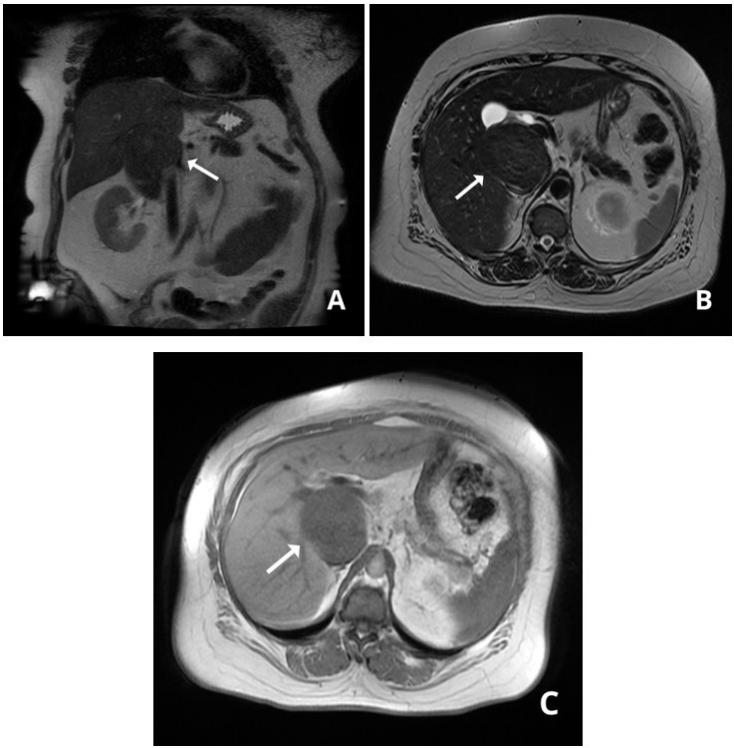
(a) Coronal magnetic resonance image in T2 showing the leiomyosarcoma exerting a mass effect on the area of the hepatic hilum and obstructing the inferior vena cava; (b) T2 axial image showing the lesion with regular outlines and well-defined limits, with low homogenous signal, and compressing the vena cava; (c) dual echo axial image showing the leiomyosarcoma in contact with segments I, V, and VI of the liver.
